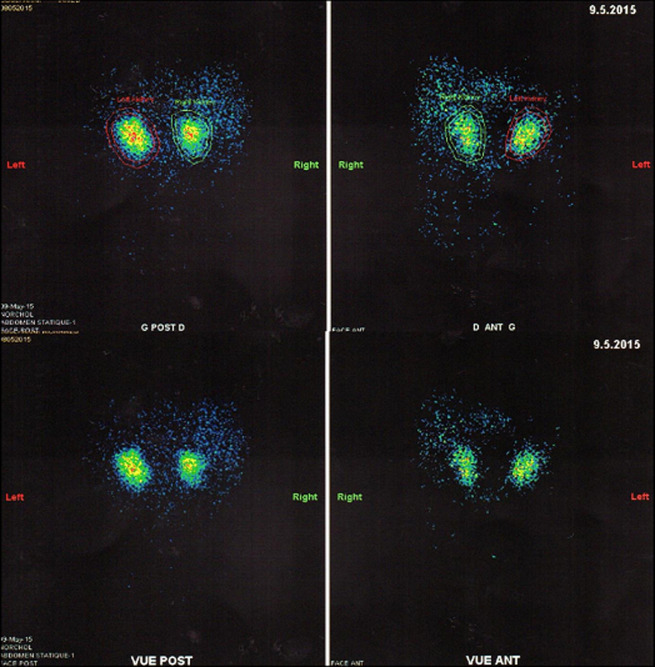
scintigraphie surrénalienne à l’iodocholestérol, montant une hyperfixation des deux surrénales en faveur d’une hyperplasie surrénalienne bilatérale, la surrénale gauche parait plus active que la droite (58% à gauche vs 42% à droite)
